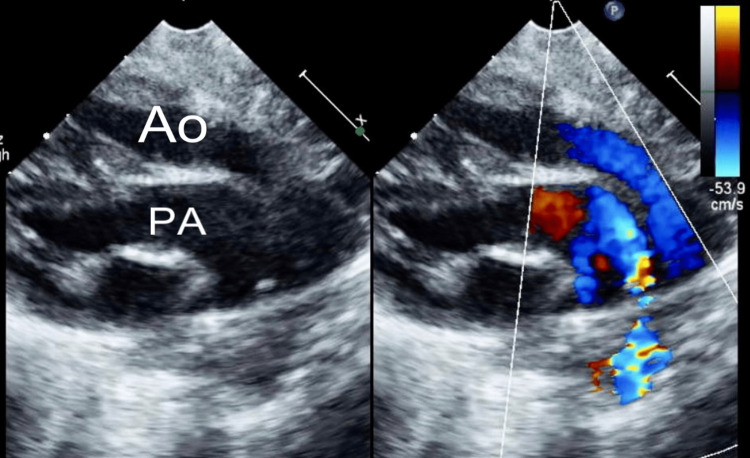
A two-dimensional Doppler echocardiogram with color flow demonstrating the great vessels with the rightward aorta (Ao) located anteriorly to the posterior pulmonary artery (PA) depicting transposition of the great arteries.
